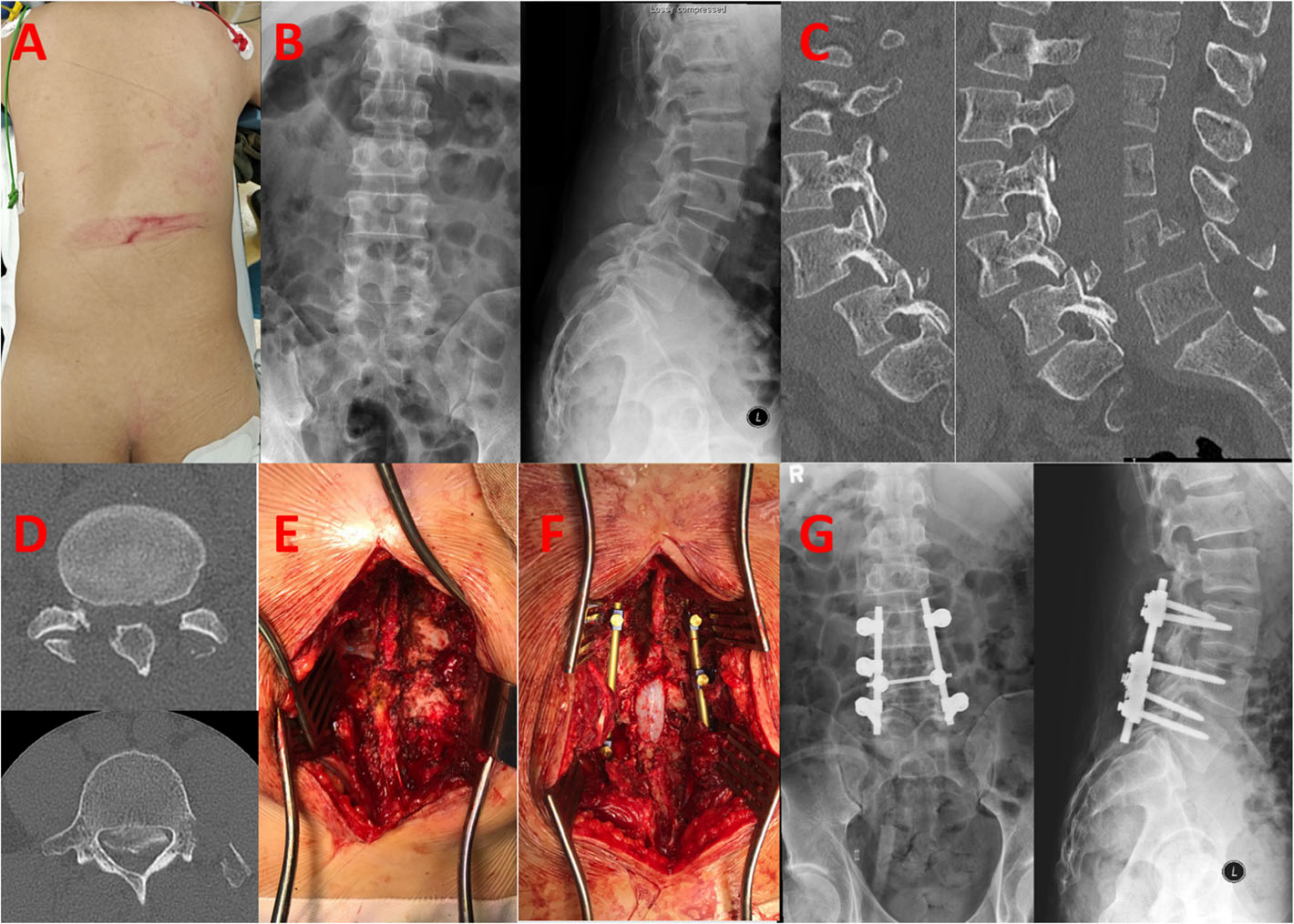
a Collision site of metal bar as fulcrum. b XR showing grade II spondylolisthesis of L4 on L5, fracture dislocation of facet joints, and fractures of left transverse processes from L1 through L4, a fracture of posterior inferior L4 vertebral body, spina bifida occulta of L5. c, d. CT scan showing fracture of bilateral L4 inferior articular processes, fracture of left L4 transverese process, left L4 pedicle and the L4 spinous process, L4 vertebral body fragment retropulsion causing severe spinal canal narrowing, and coronally oriented L4/5 facets. e Spontaneous reduction of dislocation upon prone position. f Laminectomy and posterior spinal fusion with instrumentation. g Post-operative XR showing reduction of dislocation
